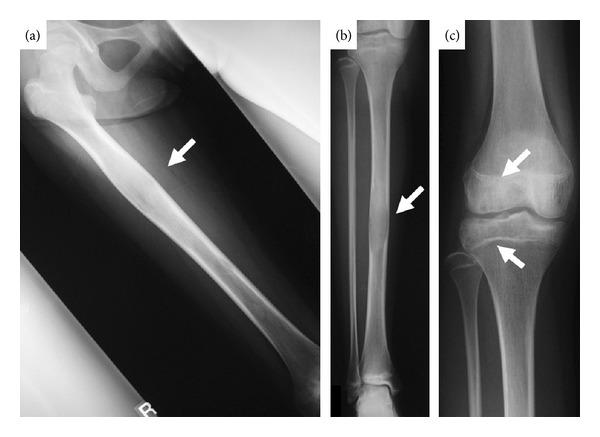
Plain radiographs of the right leg. Radiolucent lesions with sclerotic contours in the diaphyses of the right femur (a) and the right tibia (b) and the epiphyses of the right knee (c).
